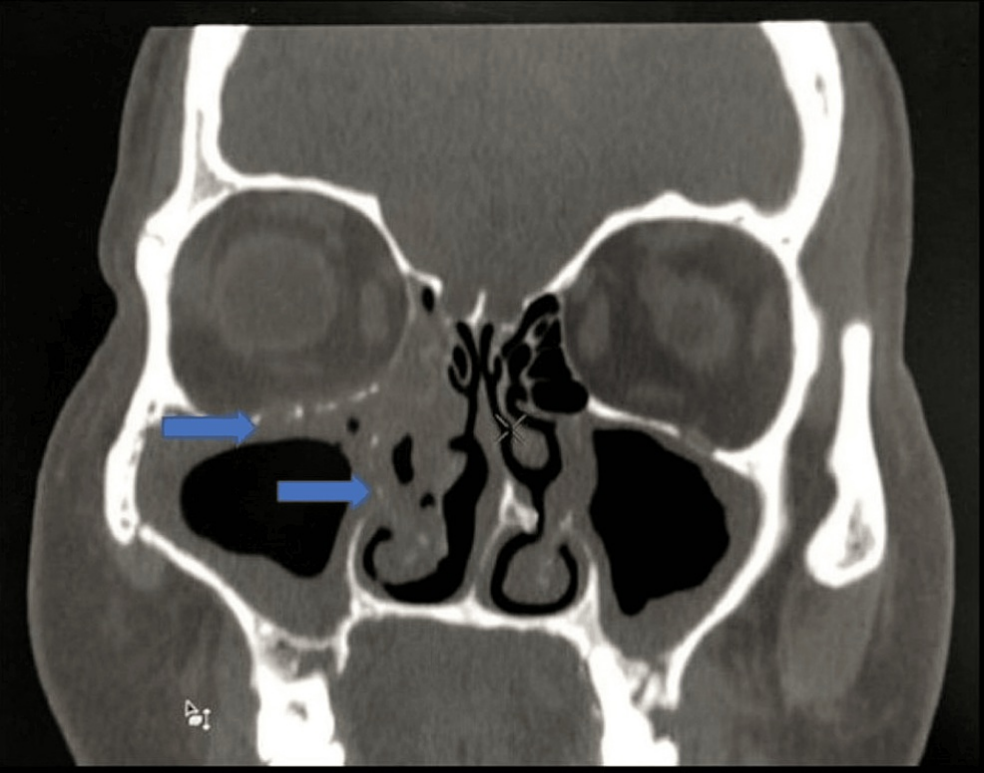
Bone involvement: case 1 The coronal section of this CT image shows mucosal thickening involving both maxillary sinuses, the right nasal cavity, and a blocked right osteomeatal complex with a permeative pattern in the inferior wall of the right orbit, the right lamina papyracea, the middle and inferior turbinate processes, and the right uncinate process, suggesting bony erosion. There is stranding in the right orbital fat involving both extraconal and intraconal compartments.
